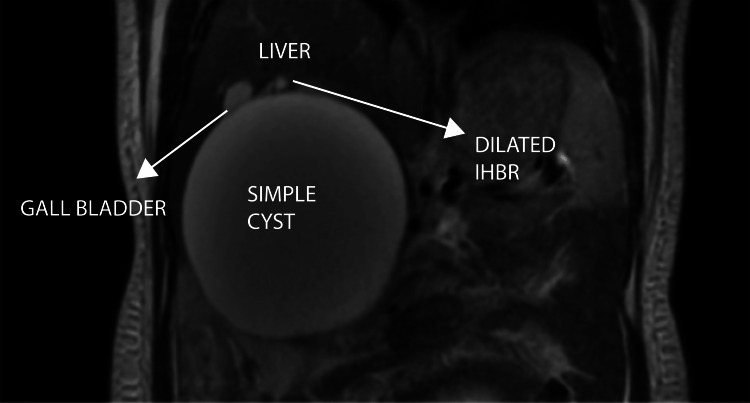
Coronal T2-weighted MRI - image 2 The image shows the lesion compressing the proximal biliary tract with mild central IHBRD. CHD and CBD could not be traced separately from the lesion MRI: magnetic resonance imaging; IHBRD: intrahepatic biliary radicle dilatation; CHD: common hepatic duct; CBD: common bile duct
